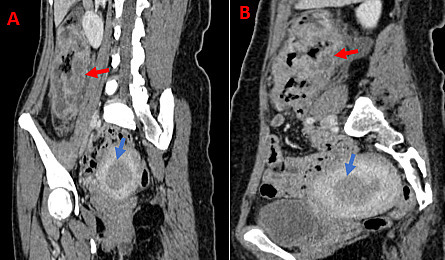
Abdominopelvic CT scan after contrast injection in coronal and sagittal oblique sections (A and B) showing thickening of the endometrium (blue arrow) associated with thickening of the right colon (red arrow)
